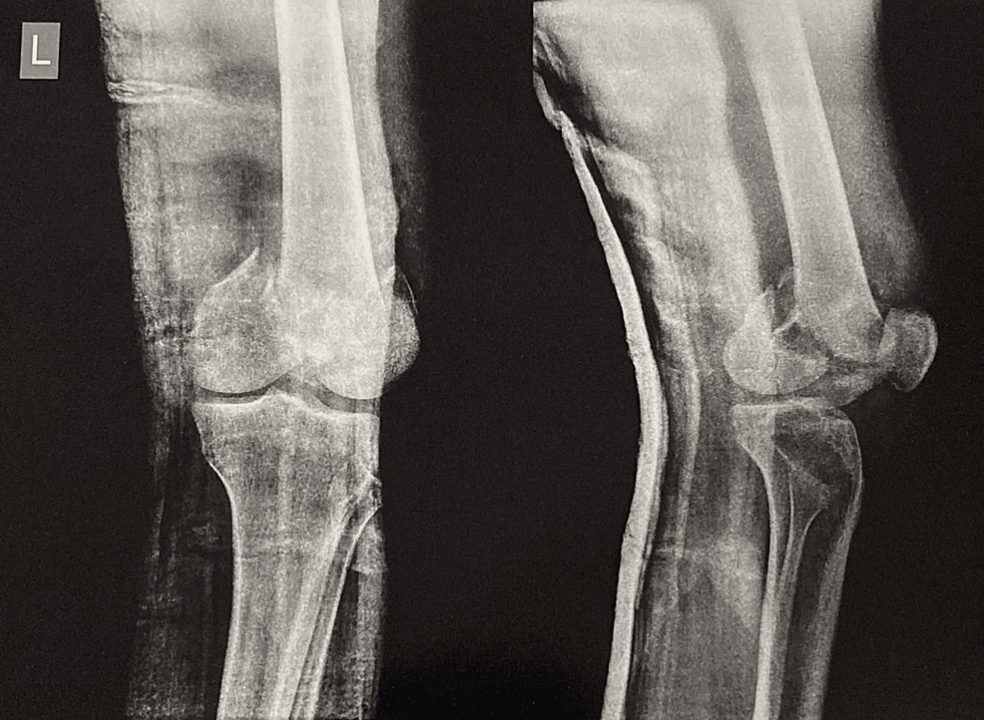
Preoperative radiograph of the knee showing distal femur fracture in anteroposterior and lateral view
